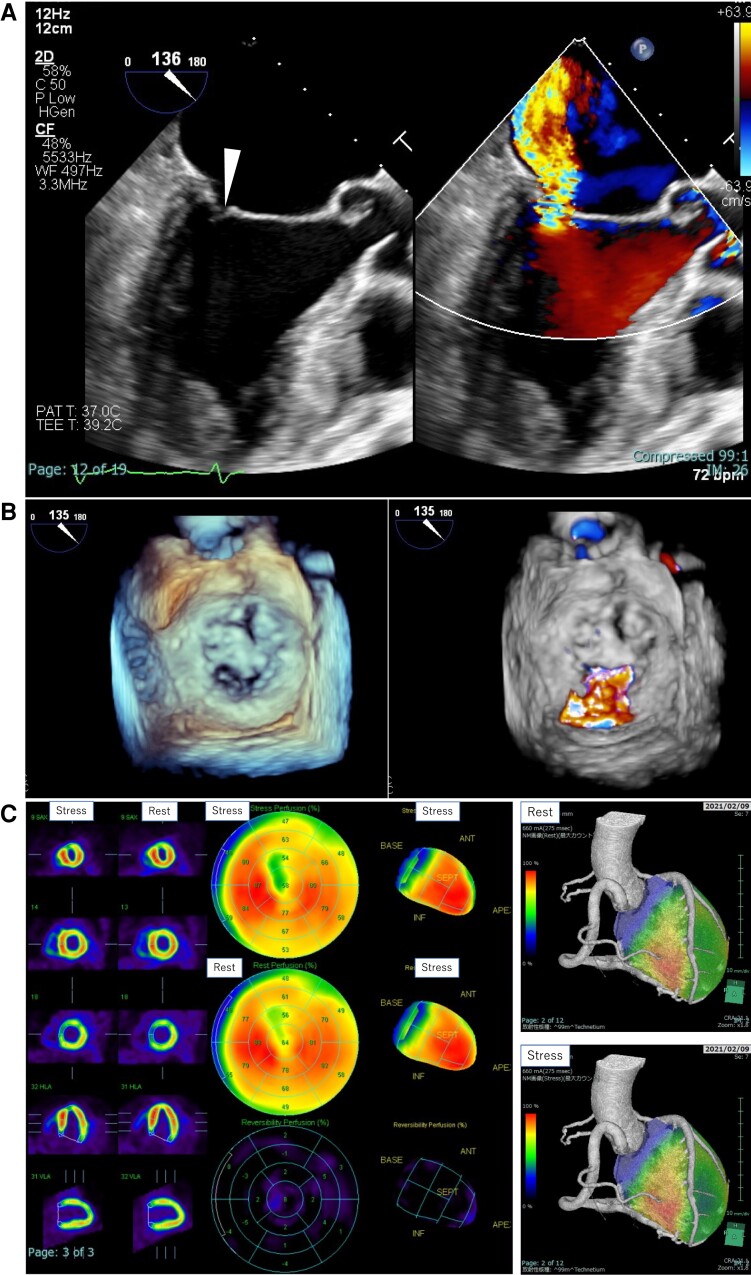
Pre-operative mitral regurgitation and myocardial perfusion. (*A*) Pre-operative transoesophageal echocardiography: long-axis view in mid-systole. Mitral annular dilatation and LA dilatation are shown. The shortened and tethered posterior leaflet and pseudo-prolapse of the anterior leaflet are shown. The arrowhead indicates the pseudo-prolapse of the anterior leaflet. (*B*) Three-dimensional (3D) echocardiography. Transoesophageal echocardiography shows a shortened and tethered mitral posterior leaflet and severe mitral regurgitation. (*C*) 99mTc-MIBI stress–rest myocardial perfusion scintigraphy. Exercise stress myocardial scintigraphy showed decreased accumulation in the antero basal-mid portion and apex, at rest and during exercise, with no myocardial ischaemia.
